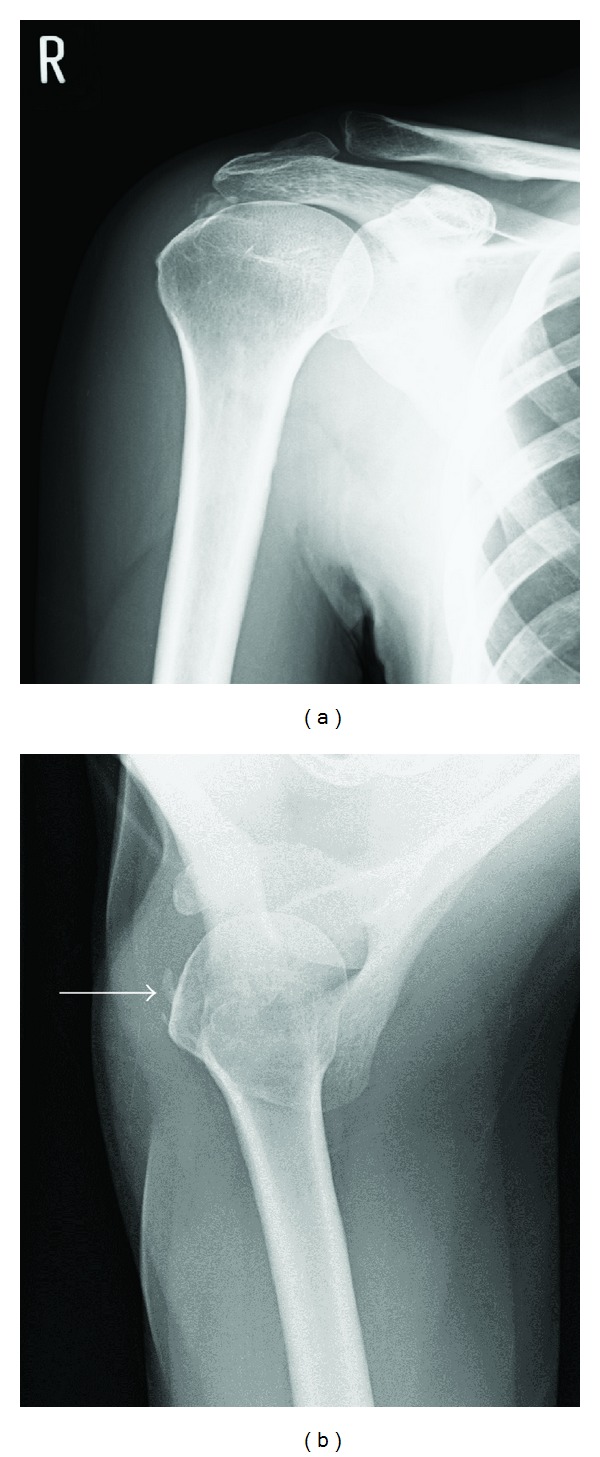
(a) and (b) Preoperative radiographs (anteroposterior view, axillary view) showing calcific deposits in the upper and anterior portion of the humerus. White arrow indicates the presence of calcific deposit at the lesser tuberosity.
